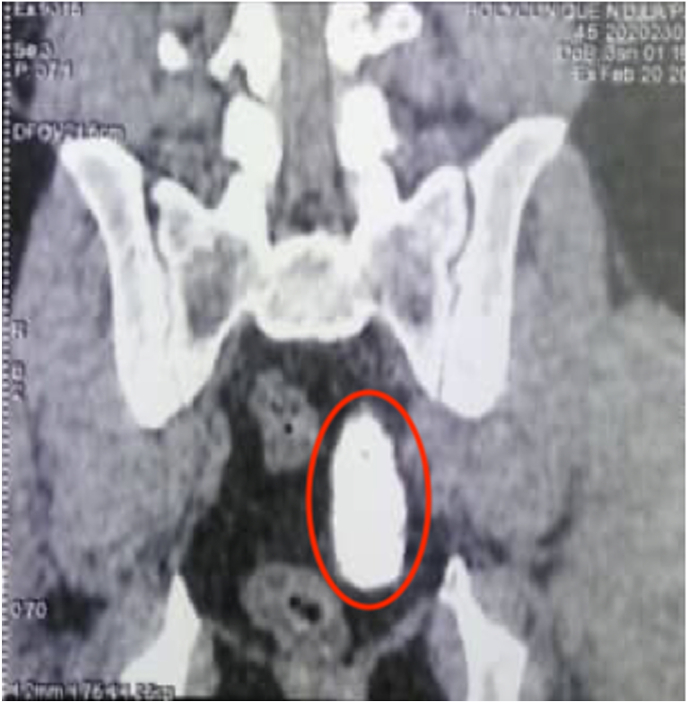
CT scan demonstrating contiguous left distal ureteric calculi circled in red measuring 22mm by 44mm.
